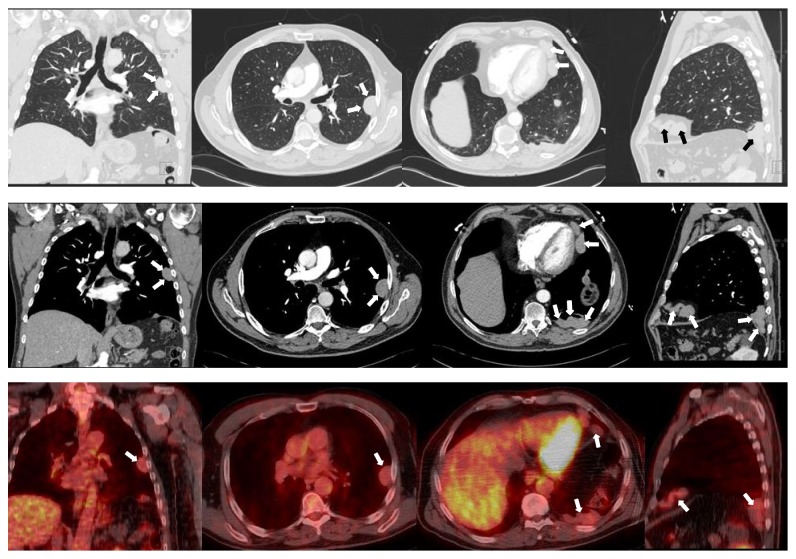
Top row: CT Chest with IV contrast, pulmonary windows. Middle row: CT Chest with IV contrast, soft tissue windows. Bottom row: fused PET/CT showing FDG uptake in the corresponding lesions. Columns (from left to right): coronal views highlighting the peripheral left mid-lung pleural-based lesion. Axial views highlighting the same peripheral left mid-lung pleural-based lesions. Axial views highlighting the pleural-based lesions at the left heart border and the posterior aspect of the left lower lobe. Sagittal views demonstrating pleural-based lesions at the anterior and posterior regions of the left hemidiaphragm.
